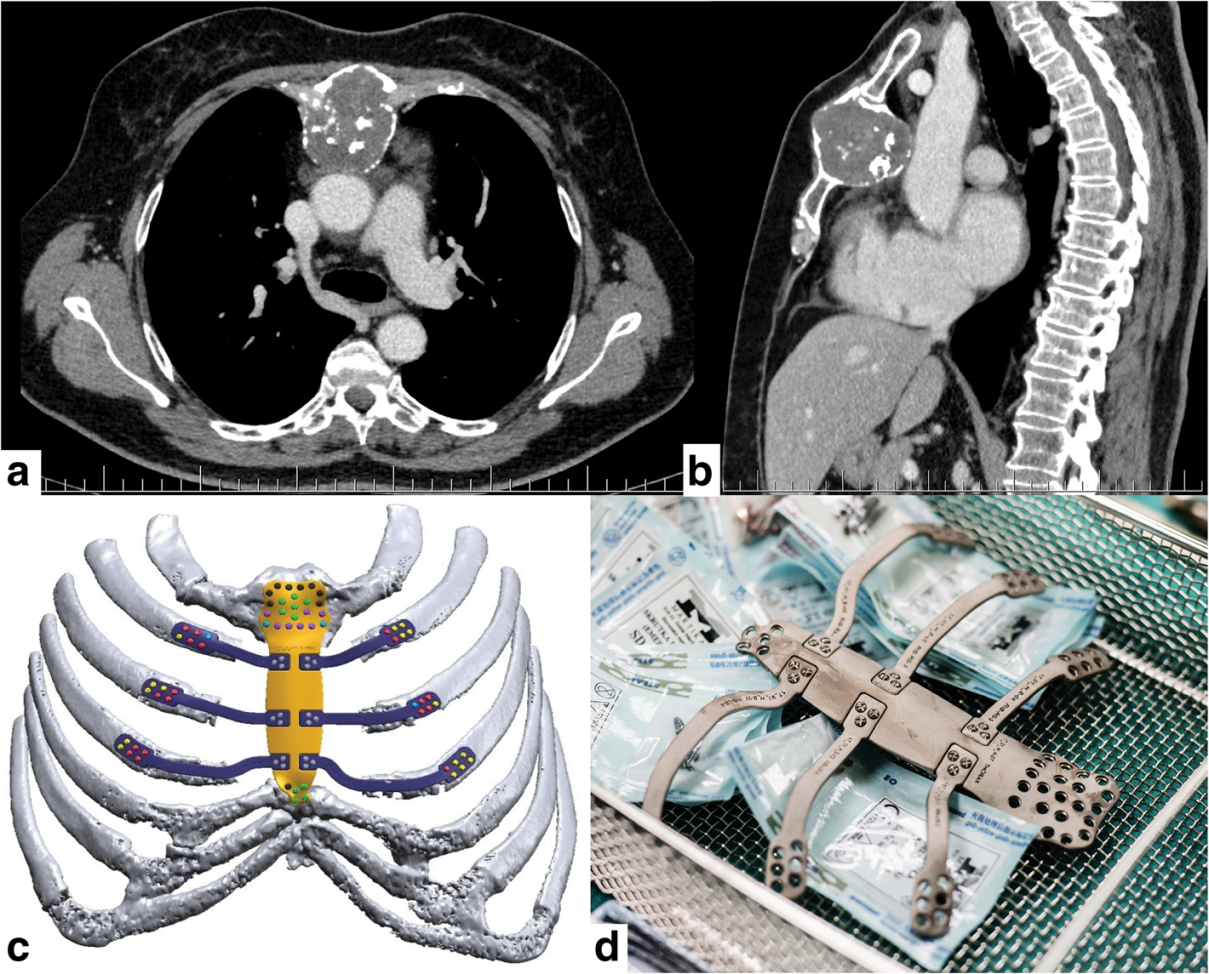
a Axial chest computed tomography of the sternal tumor. b Sagittal computed tomographic reconstruction of the sternal tumor. c Digital model of the implant. d Titanium implant ready for insertion
